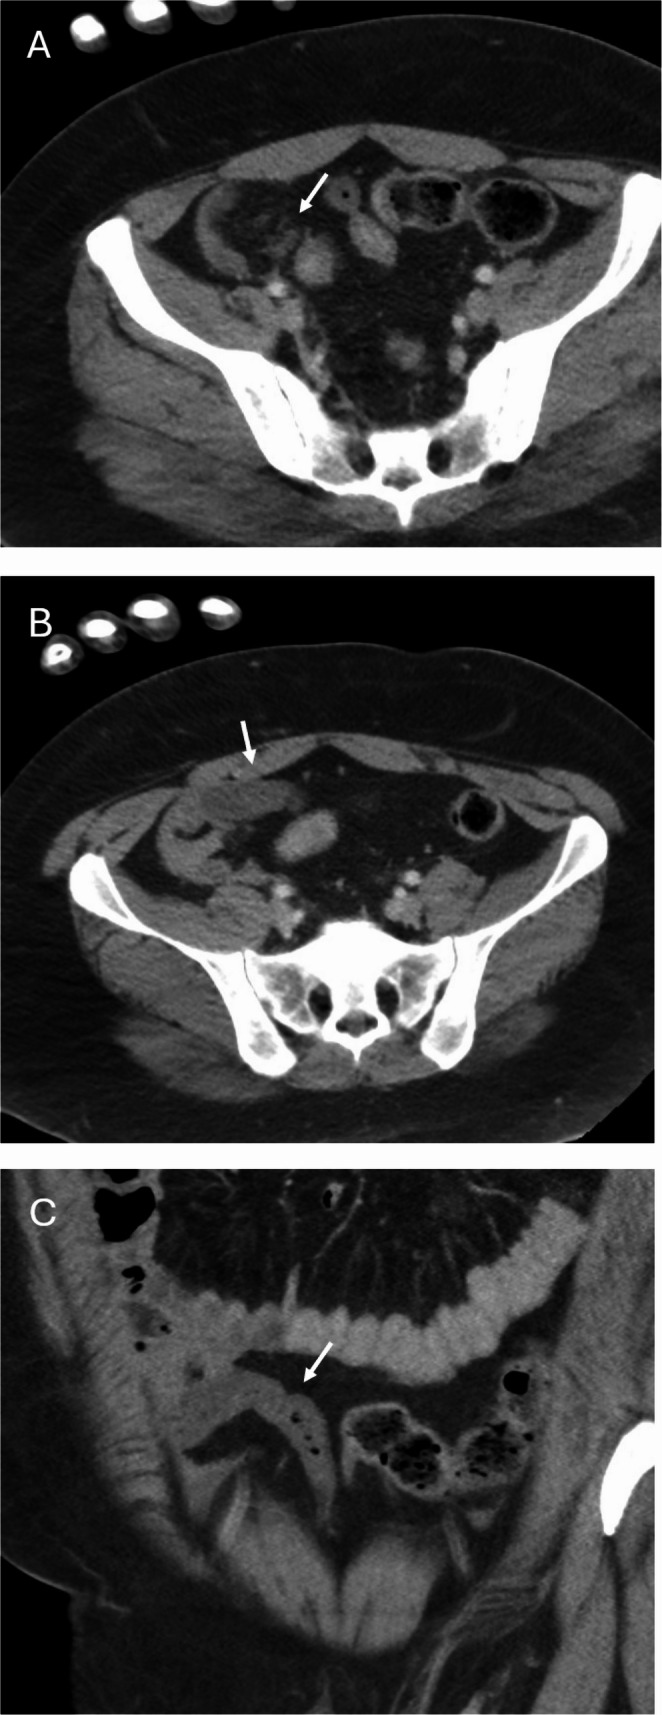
27-year-old male with abdominal pain status-post motor vehicle collision. Axial (A, B) and coronal (C) postcontrast CT images through the pelvis demonstrate a subtle focus of mesenteric fat stranding (A, arrow) in the right lower quadrant indicative of a mesenteric injury, which was not described on the original outside report. There is an adjacent loop of poorly enhancing small bowel (B & C, arrows) which also was not described on the original outside report. At surgery, there was a small bowel mesenteric bucket handle injury associated with devitalized small bowel. A total of 30 cm of small bowel was resected.
